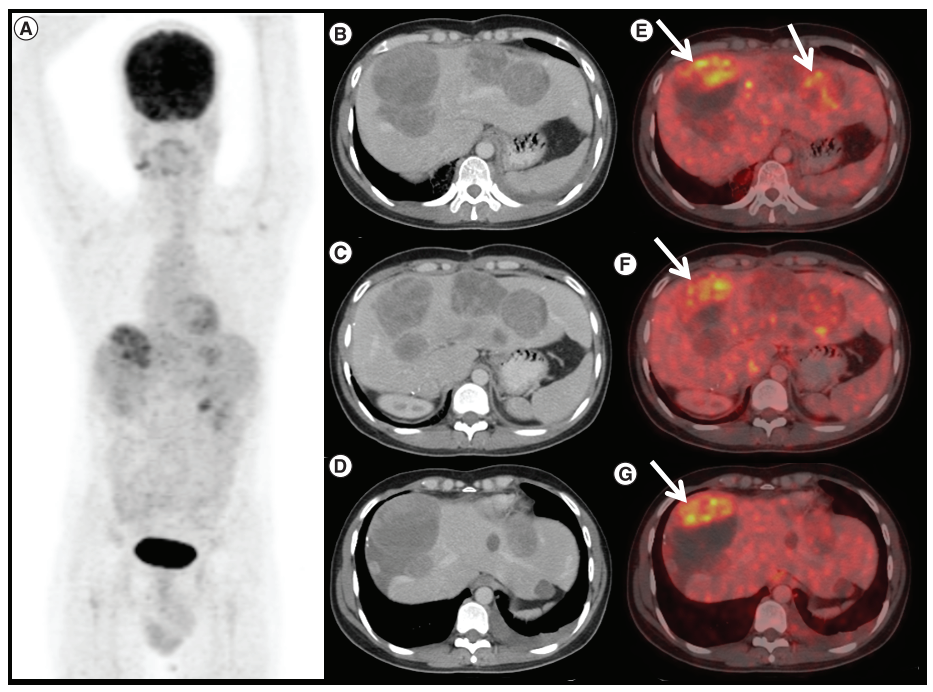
Response to avapritinib therapy in case 1. ^1^^8^F-FDG positron emission tomography/computed tomography (PET/CT) done 6 months after avapritinib therapy in patient A. (A) Maximum intensity projection, (B, C & D) axial contrast enhanced CT and (E, F & G) fused PET/CT images shows increase in size of the liver lesions with FDG avid enhancing areas in the periphery of the lesion (arrows), suggestive of progressive disease.
